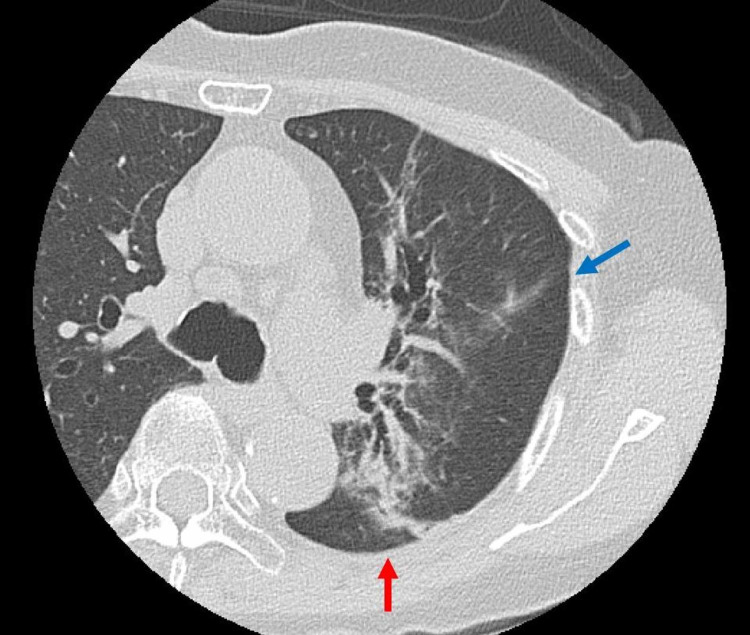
CT image 1.5 months prior to COVID-19 infection. The red arrow shows radiation fibrosis and the blue arrow shows interlobar fissure. CT, computed tomography; COVID-19, coronavirus disease 2019
